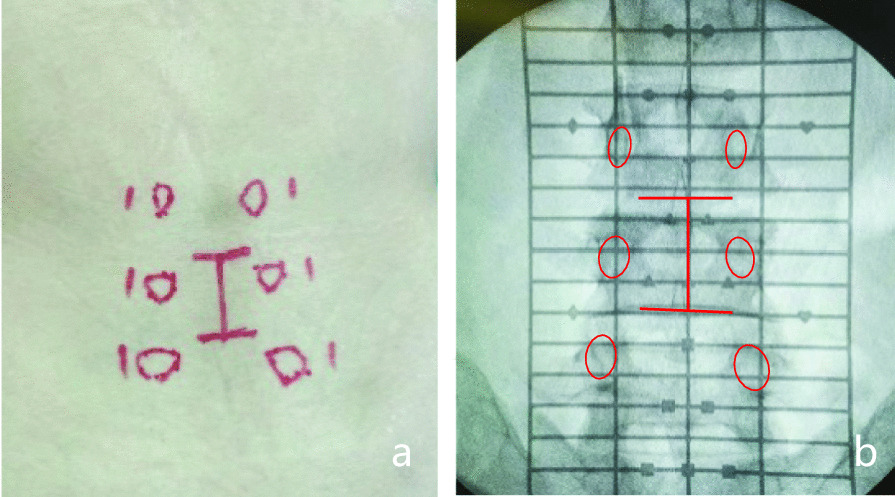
Multilevel mark line on skin. a The intervertebral space and projection of pedicle were determined under C-arm fluoroscopy and marked. Then, mark the surgical incision along the spinous process between the two intervertebral spaces and 1.5 cm lateral to the pedicle mark the puncture points of PPS. b Corresponding mark position on X-ray
